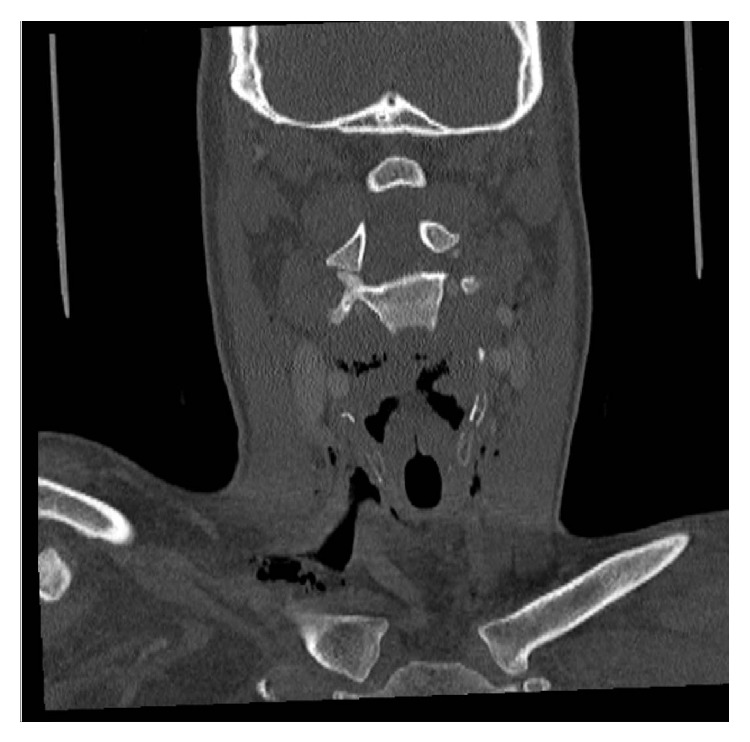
Computed tomography showing extensive surgical emphysema in the deep neck tissues.
